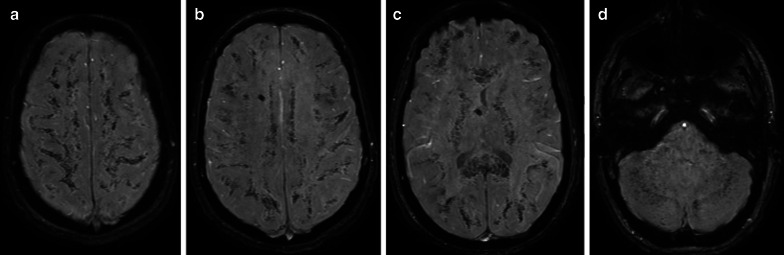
A case representing the walnut kernel microbleed pattern. SWI (a–d) MRI images at the level of the centrum semiovale (a), at the level of the corona radiata (b), at the level of the basal ganglia (c), and at the level of the brainstem and the cerebellum (d) of a 16-year-old polytraumatised female patient acquired on day 12 after hospital admission. SWI shows very high number of monotonous punctuate microbleeds in the subcortical white matter, the internal capsule, the corpus callosum, the cerebellum and the brainstem resembling a “walnut kernel”. The larger hypointensity visible in the right centrum semiovale and near the third ventricle is due to a ventricular drain (a–c)
